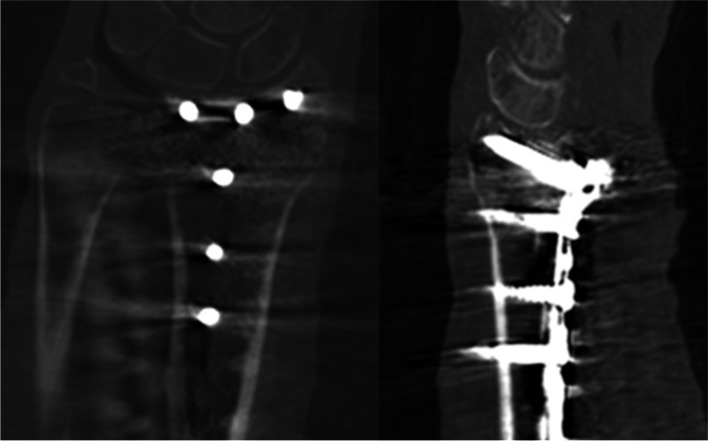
CBCT images in coronal and sagittal planes
